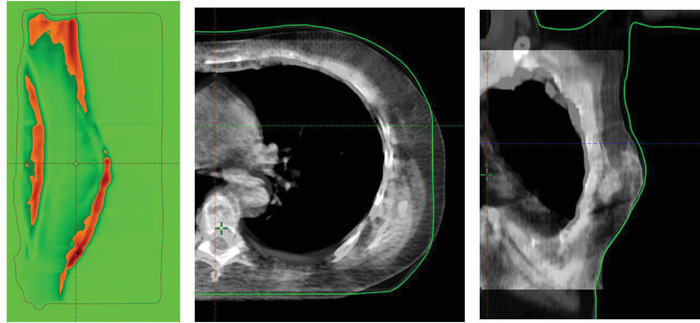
Eighth fraction of patient 3. Differences in passing rate are in the chest wall and the outer contour of the breast. CBCT images show differences in the same areas
